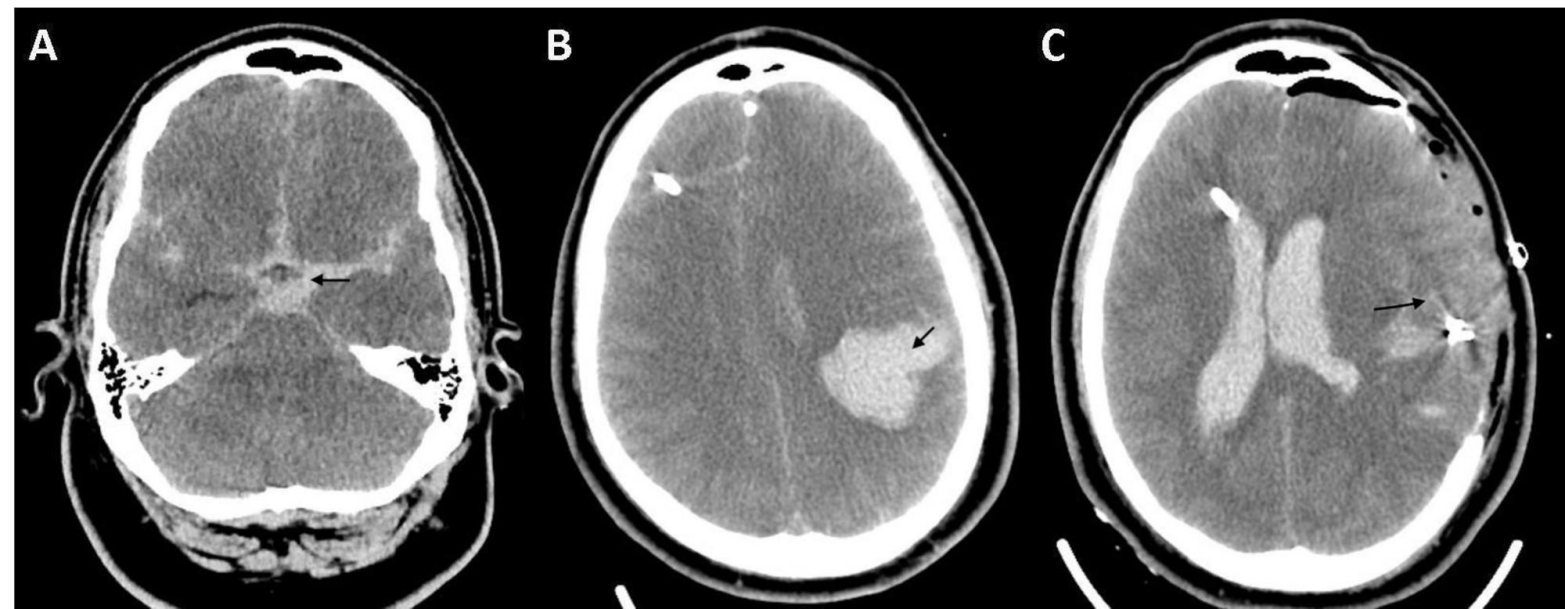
Computed tomography (CT) of the head (A) At admission: Subarachnoid hemorrhage (SAH) at the basal cisterns; (B) Six hours post-admission: left intracerebral hemorrhage was noted; (C) Two days post-decompressive craniectomy: hyperdensity in surgical bed and clips. On the left, the intraventricular hemorrhage is seen in the lateral ventricle with the external ventricular drain (EVD) in the right frontal horn.
